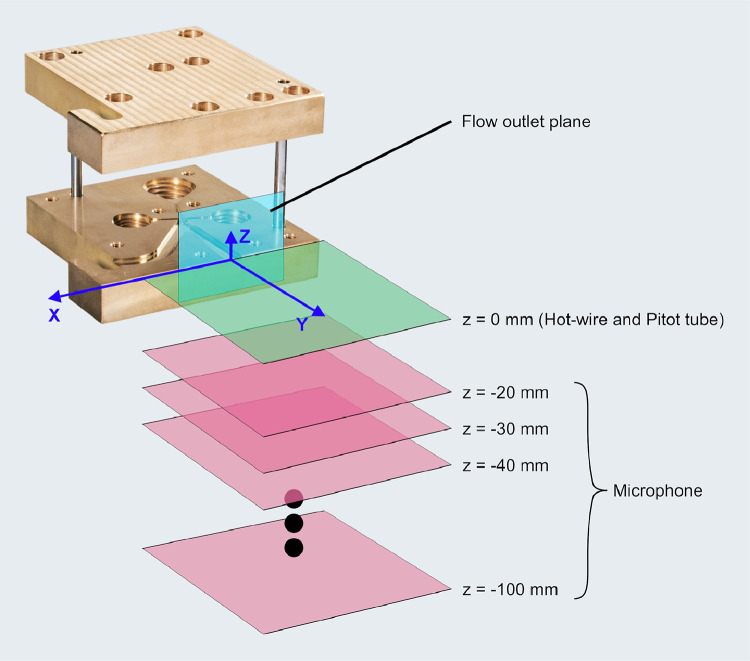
Measurement planes downstream of the fluidic ultrasonic transducer. For the piezoelectric transducer the flow outlet plane coincides with the actuation plane. The figure has been adapted from the accompanying study [1], with the measurement planes being added for this publication.
